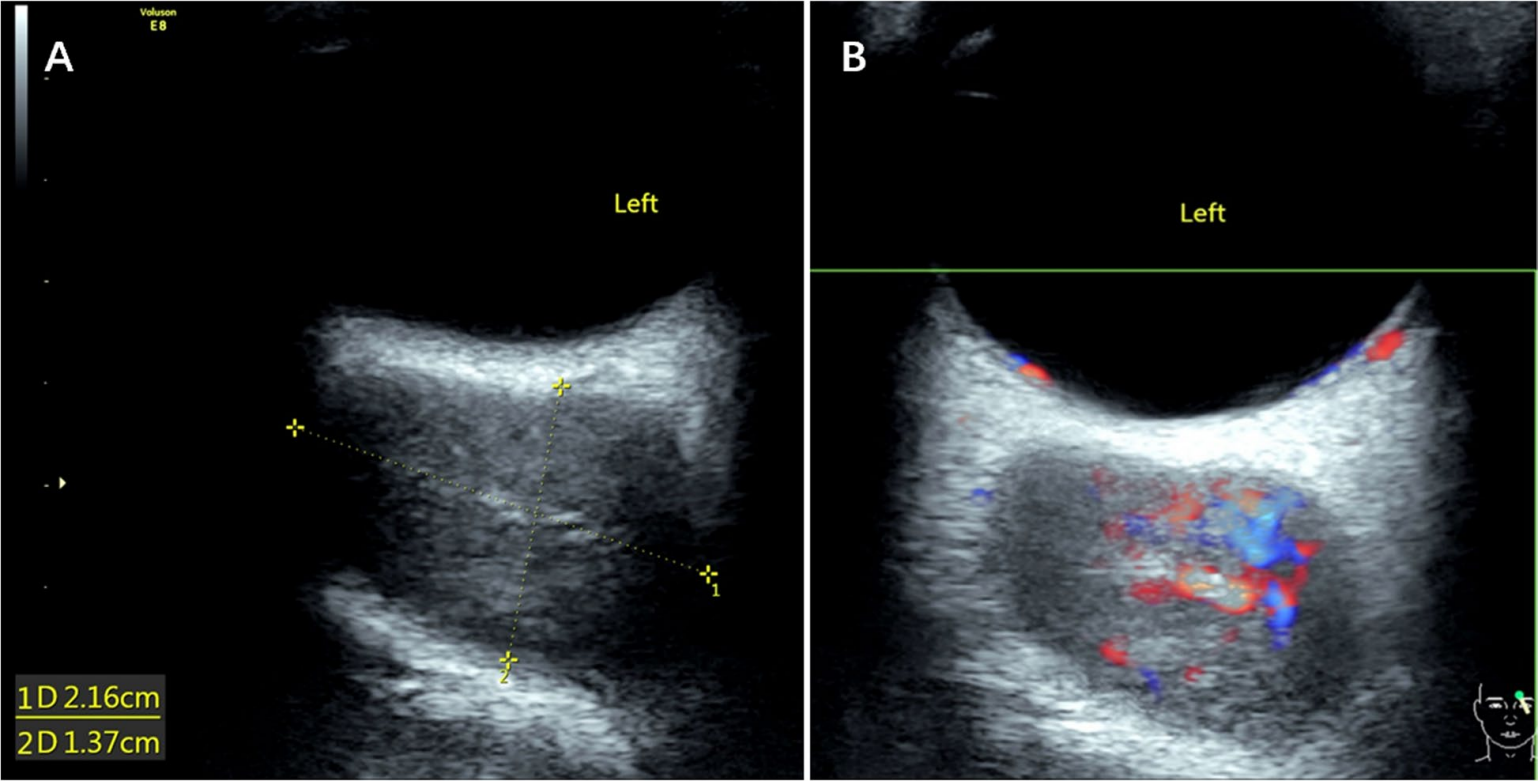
The left orbital colour Doppler ultrasound. The orbital subnasal lesion mass is in the size of 2.16*1.37 cm, and has a heterogeneous hypoechoic-isoechoic appearance; the blood flow is relatively rich
